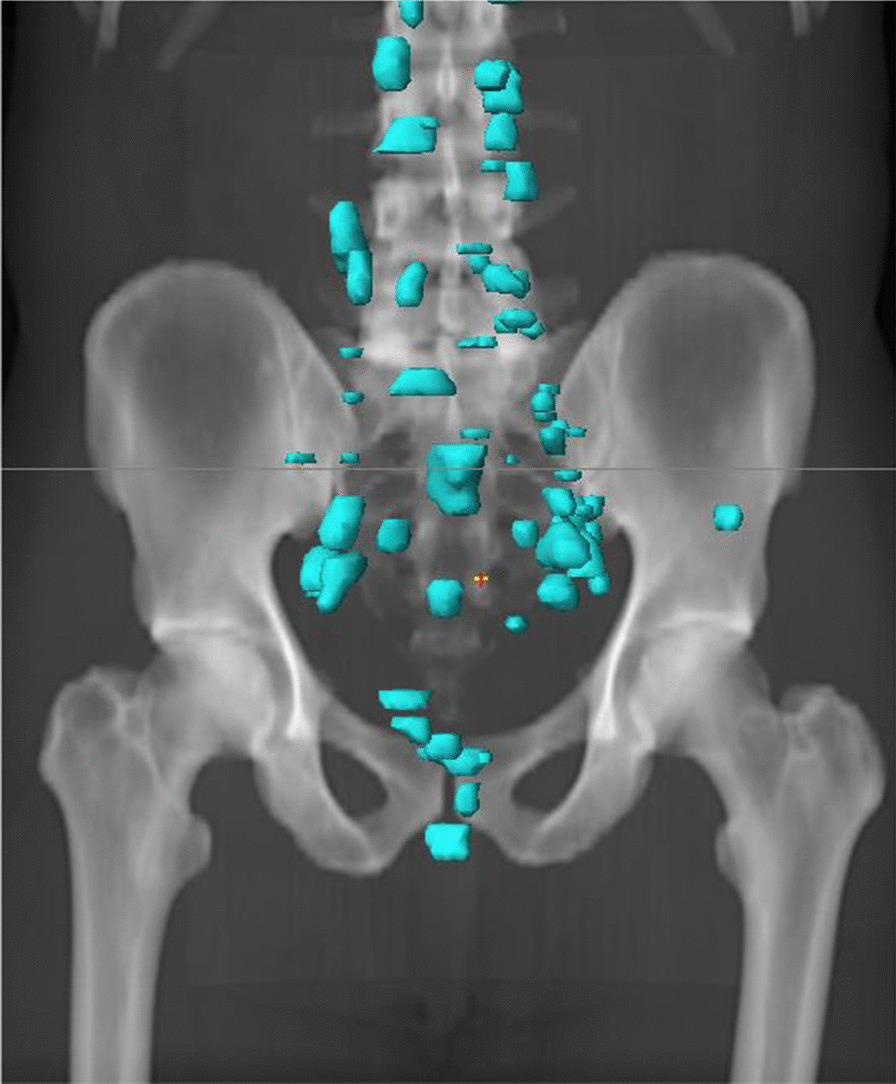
Distribution of ^18^F-PSMA PET/CT avid lesions in the abdomen and pelvis (pulmonary and supraclavicular metastases not shown) for the 32 patients included in the analysis
